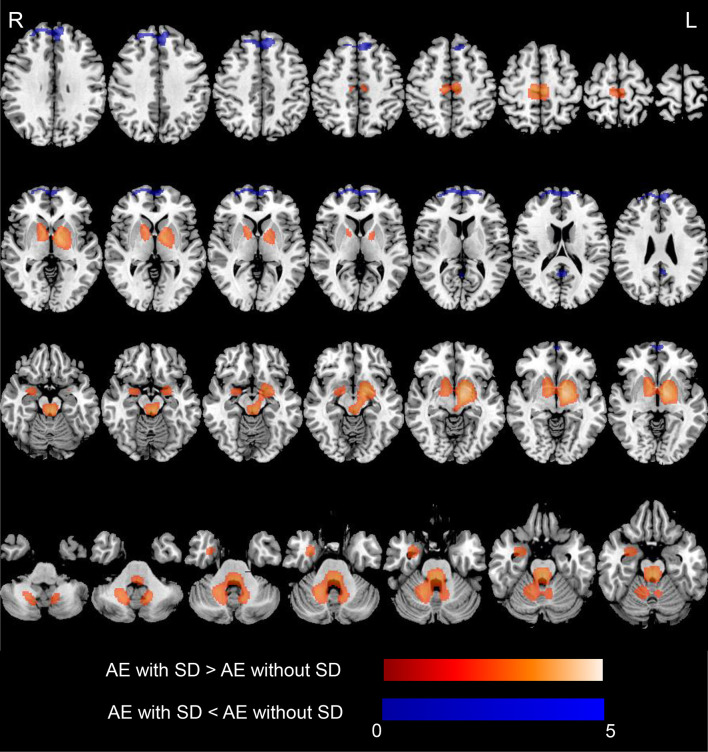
^18^F-FDG-PET results based on SPM in AE patients with SD compared with those who did not develop SD. T-maps show hypo- (blue color) and hyper-metabolism (hot color). Patients with SD demonstrated relatively increased metabolism in the MTL (amygdala, hippocampus), and basal ganglia (medial globus pallidus, putamen, caudate), extending to the cerebellum (cerebellum anterior lobe, cerebellum posterior lobe, dentate, cerebellar tonsil, culmen), brainstem (midbrain, pons), and thalamus and hypothalamus (*p* < 0.05, FDR corrected). Patients with SD showed hypometabolism in the frontal lobe (medial frontal gyrus, superior frontal gyrus), posterior cingulate cortex (*p* < 0.001, uncorrected). Axial slices are shown according to radiological convention (right is left). ^18^F-FDG-PET, ^18^F-fluorodeoxy-glucose positron emission tomography; SPM, statistical parametric mapping; AE, autoimmune encephalitis; SD, sleep disorders; R, right; L, left.
